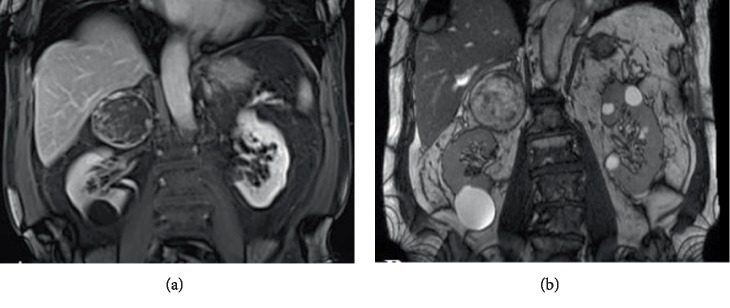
Enhanced magnetic resonance images (MRI) of the abdomen and pelvis with gadolinium. (a) Coronal view of an in-phase T1-weighted image identifying a large suprarenal mass measuring around 7.3 × 6.5 × 6 cm occupying the space of the right adrenal gland, showing a significant rim enhancement with marked vascularity. (b) Coronal view of a T2-weighted image identifying the similar right suprarenal mass with heterogeneous component inside it, possessing various signal intensities.
